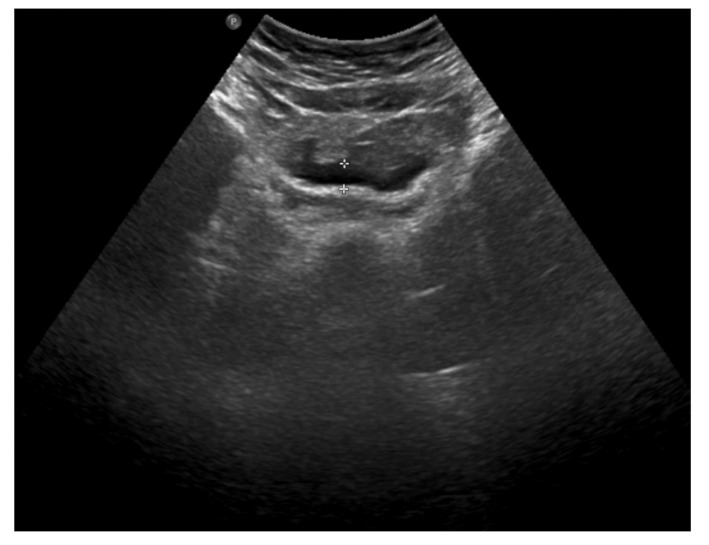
The abdominal ultrasound on the seventh day of treatment: partial regression of fluid collections and hyperechoic adipose tissue lesions.
